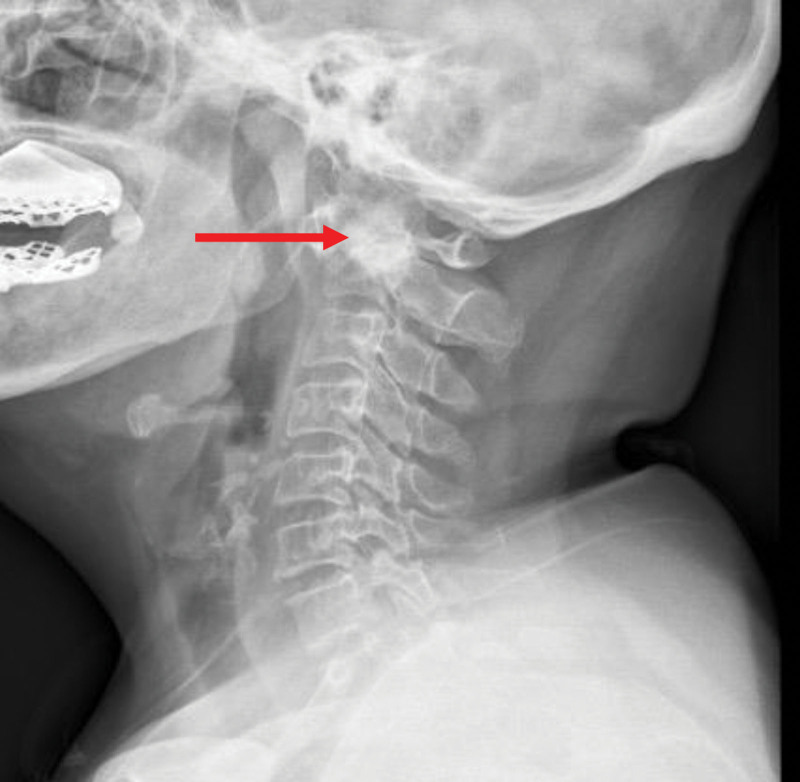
Cervical spine X-ray (lateral view): Atlantoaxial joint space narrowed.
